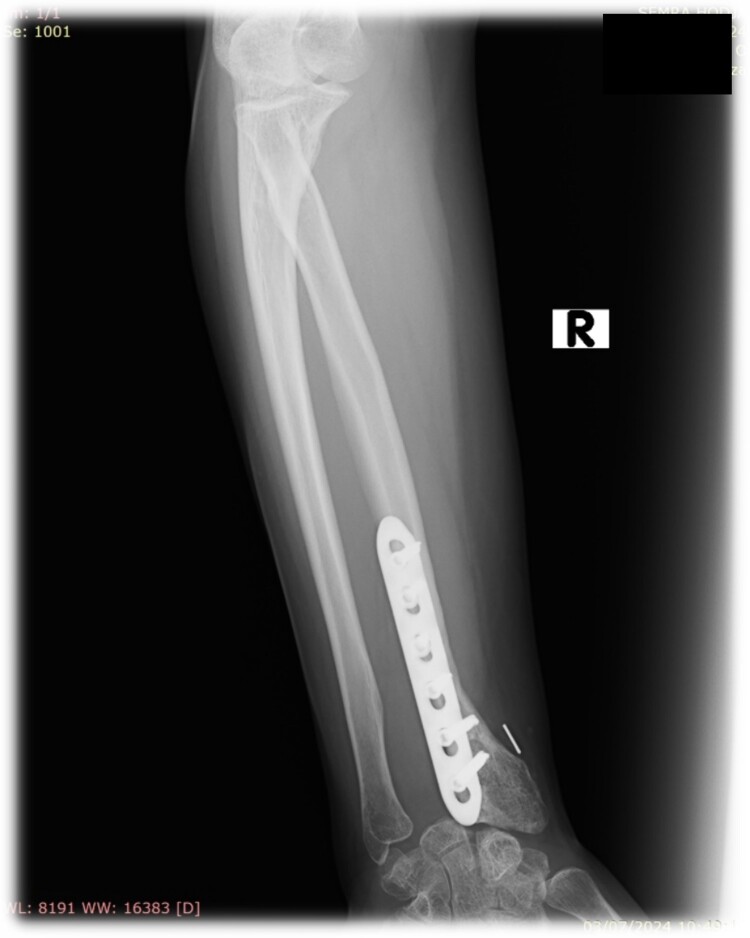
Follow-up X-ray of the right forearm 2 years after the surgery in anterior–posterior plane.
